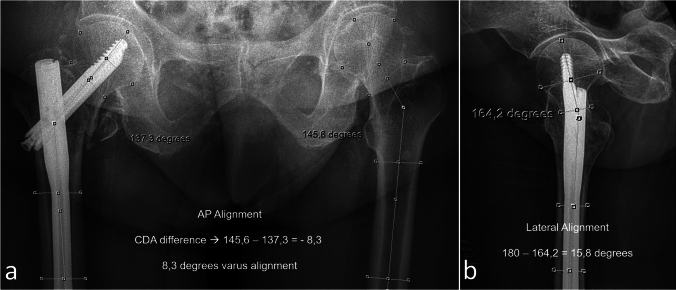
Evaluation of fracture alignment in AP and lateral planes. The difference in collo-diaphyseal angle compared to the intact hip on AP radiograph (a). Angulation between the diaphyseal axis and the head-neck axis in the lateral plane (b)
